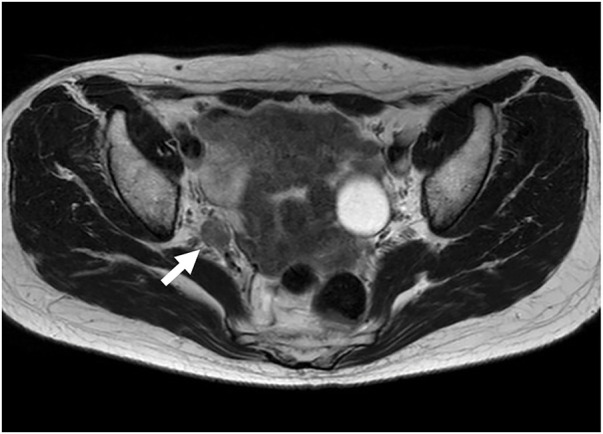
MRI finding. MRI scan showed enlargement of the right lateral lymph node (white arrow), whose region was distal of the internal iliac artery, and whose diffusion-weighted images showed a high signal.
